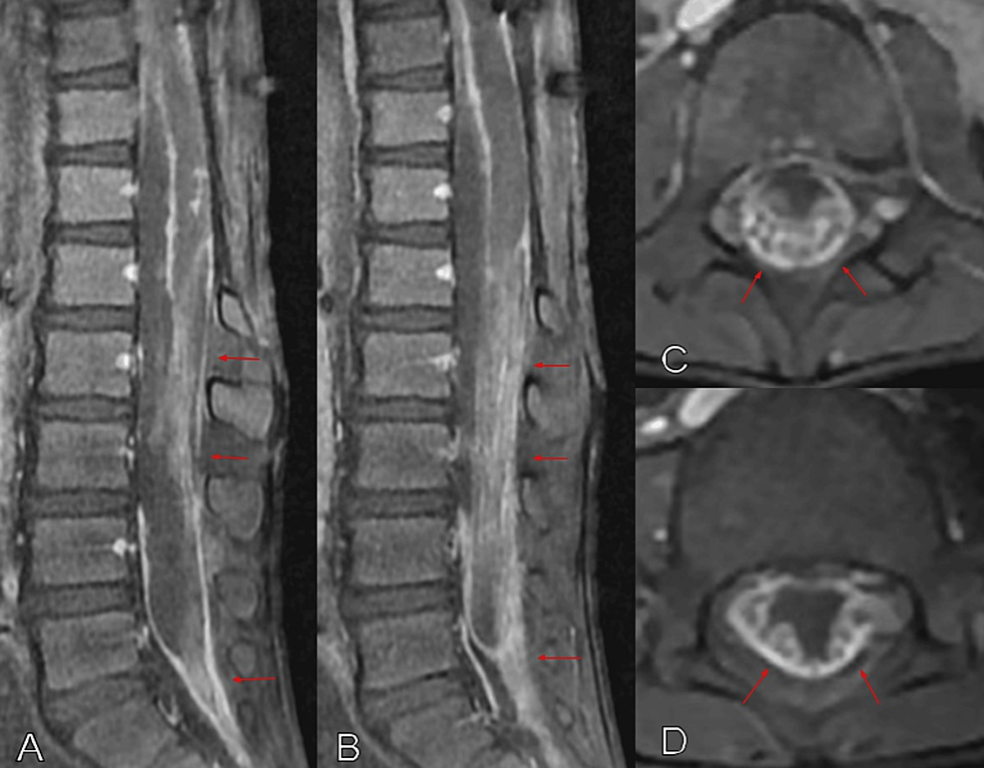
Lumbar MRI in sagittal (A, B) and axial (C, D) T1 views with gadolinium showing the extensive leptomeningeal spread of disease throughout the spine (red arrows).
